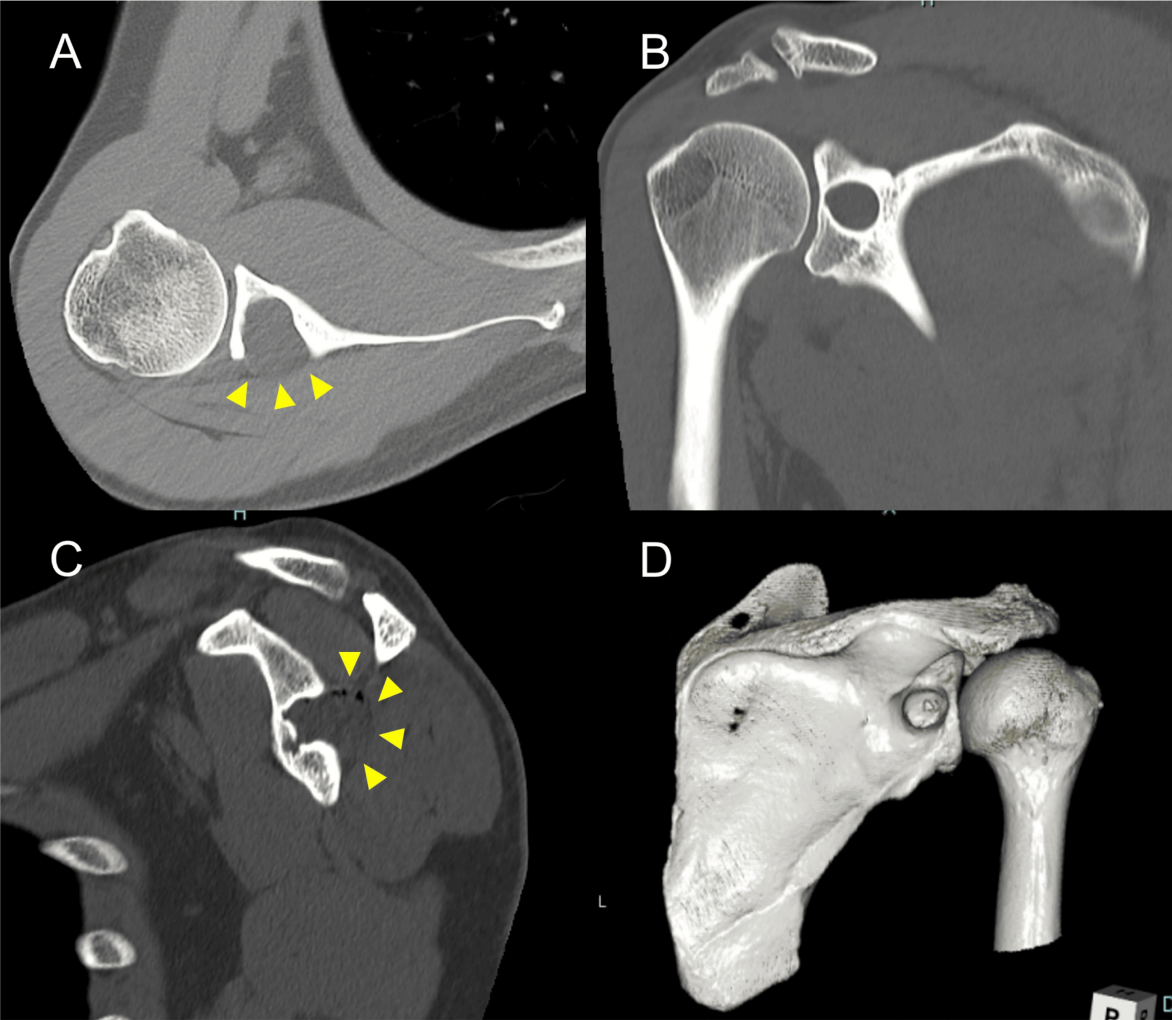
Preoperative right shoulder computed tomography (CT) images The preoperative (A) axial, (B) coronal, (C) sagittal, and (D) posterior three-dimensional (3D) views of CTs show a bony defect area at the mid-portion of the glenoid containing a cystic lesion (triangle heads).
